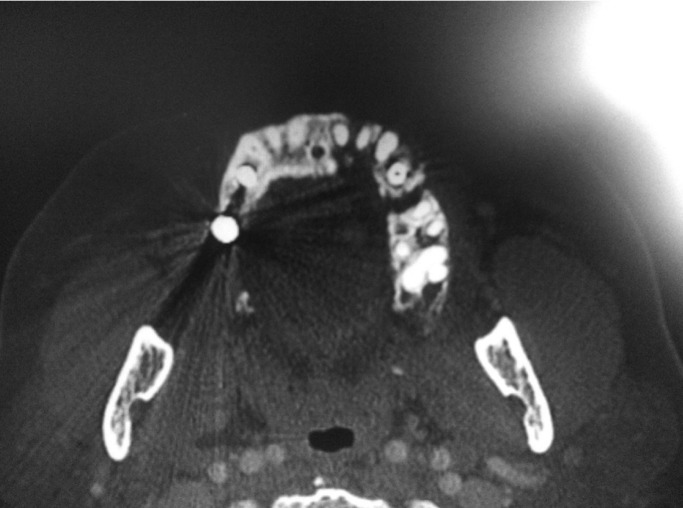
CT shows left side maxillary osteonecrosis.
